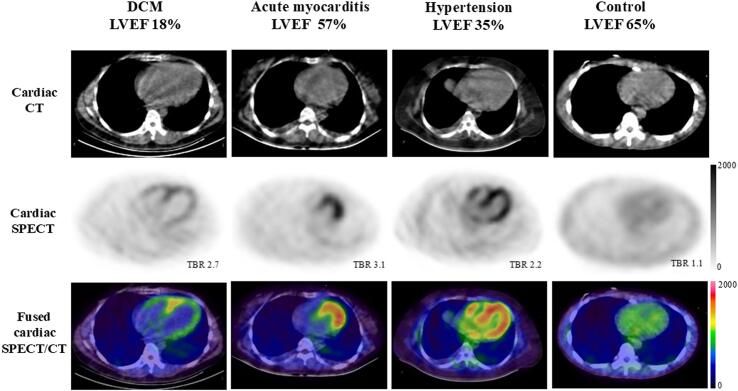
Detailed ^99m^Tc-HFAPi images of the patients with non-ischemic HF and control. CT (first row), SPECT (second row), and fused SPECT/CT (third row) images in patients caused by different etiologies (DCM [first column], acute myocarditis [second column], hypertension [third column]) and control (fourth column). Intense but heterogeneous ^99m^Tc-HFAPi uptake was observed in the LV myocardium of patients, whereas ^99m^Tc-HFAPi uptake was not observed in the control. ^99m^Tc-HFAPi, ^99m^Tc-labeled–hydrazinonicotinamide-fibroblast activation protein inhibitor-04; SPECT/CT, single photon emission computed tomography/computed tomography; DCM, dilated cardiomyopathy; LV, left ventricle.
